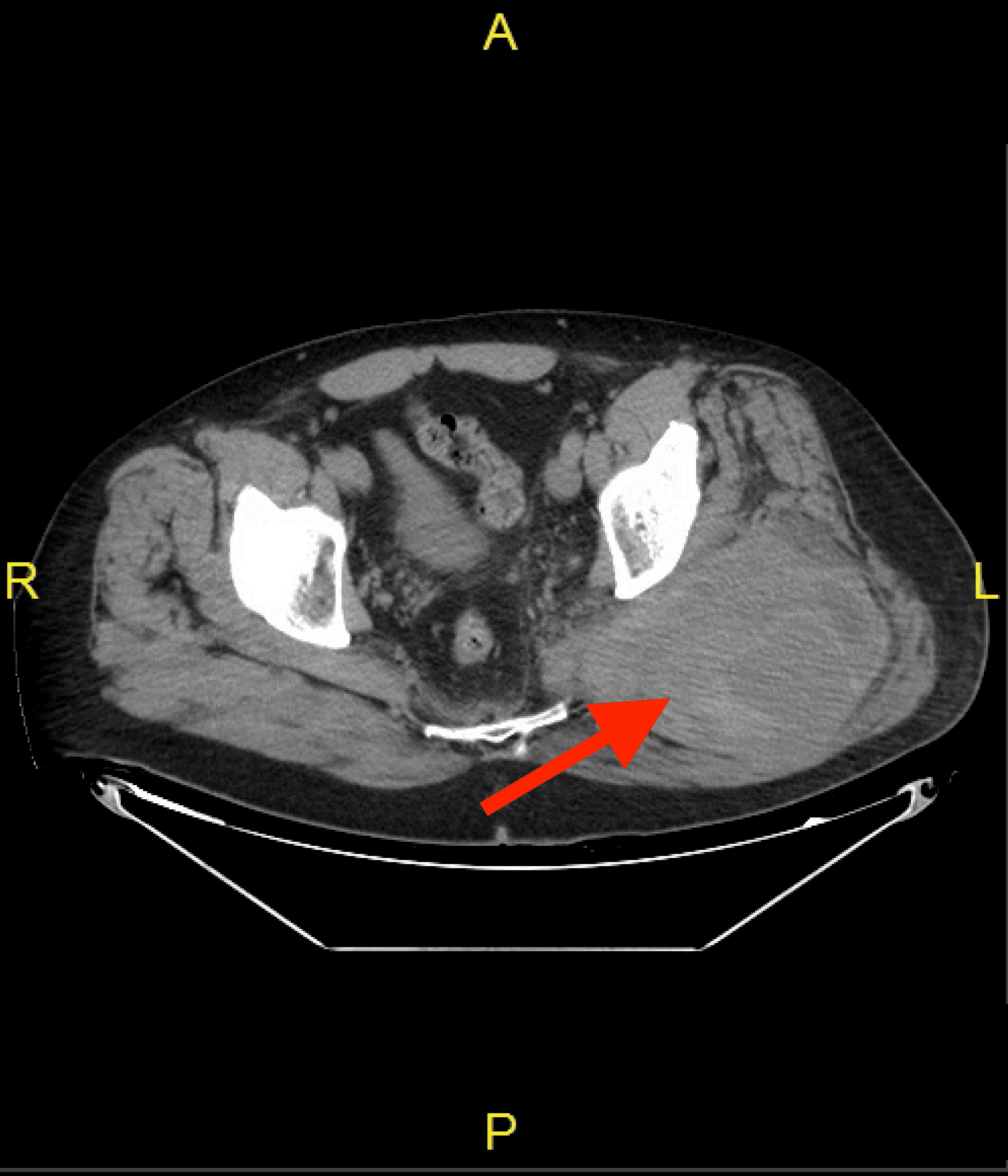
CT of the pelvis (axial view) No concerning bony lesions were identified. The left gluteal soft tissue has an enlarging, heterogeneous fluid collection (red arrow), approximately 10.8 x 14.6 x 15 cm, representing an expanding hematoma with blood pooling on venous phase imaging concerning active extravasation.
